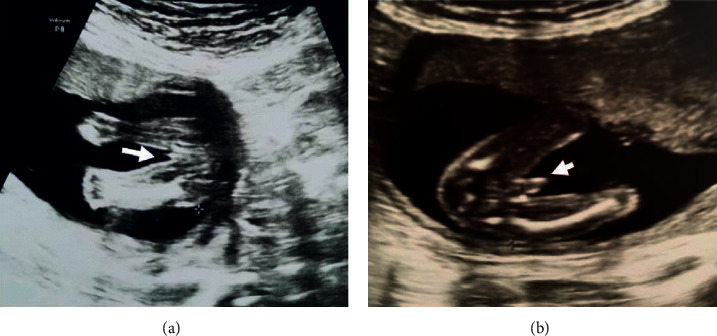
Examples of fetal ultrasound images showing transverse views of a 20-week female fetus (a) and an 18-week male fetus (b) demonstrating labial folds and phallus, respectively (arrowheads).
